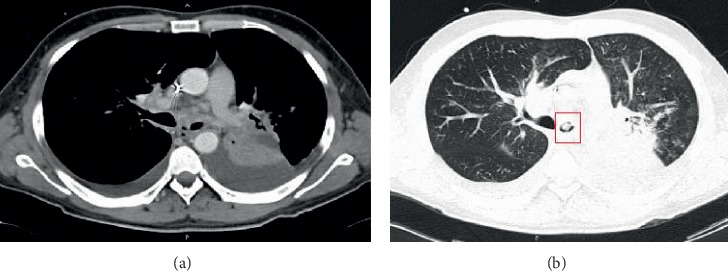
(a) Thorax CT showing a dense consolidation in the lower lung left lobe with mass aspect and pleural effusion. (b) Tumor obstructing 90% of the left main bronchus (red box).
